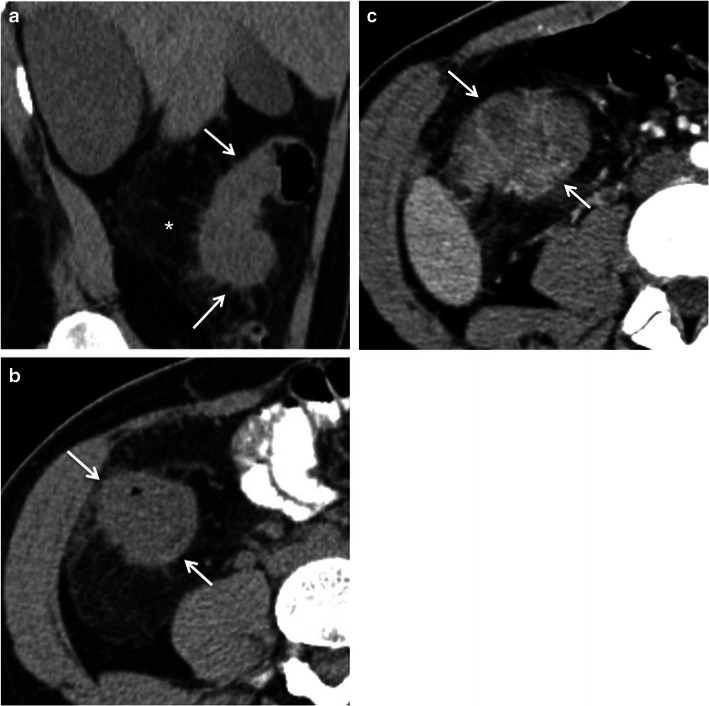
Crohn’s disease mimicking colon cancer. Reformatted coronal (a) and axial (b) unenhanced CT scans and axial contrast-enhanced CT scan (c) show spiculated irregular wall thickening of the caecal wall (*arrow* in a–c) with heterogeneous contrast enhancement (c). Also note proliferation of the pericaecal fat (*asterisk*), a common finding in Crohn’s disease. *Adapted from the electronic poster “Bowel wall thickening—a complex subject made simple” DOI:*10.5444/esgar2011/EE-063
